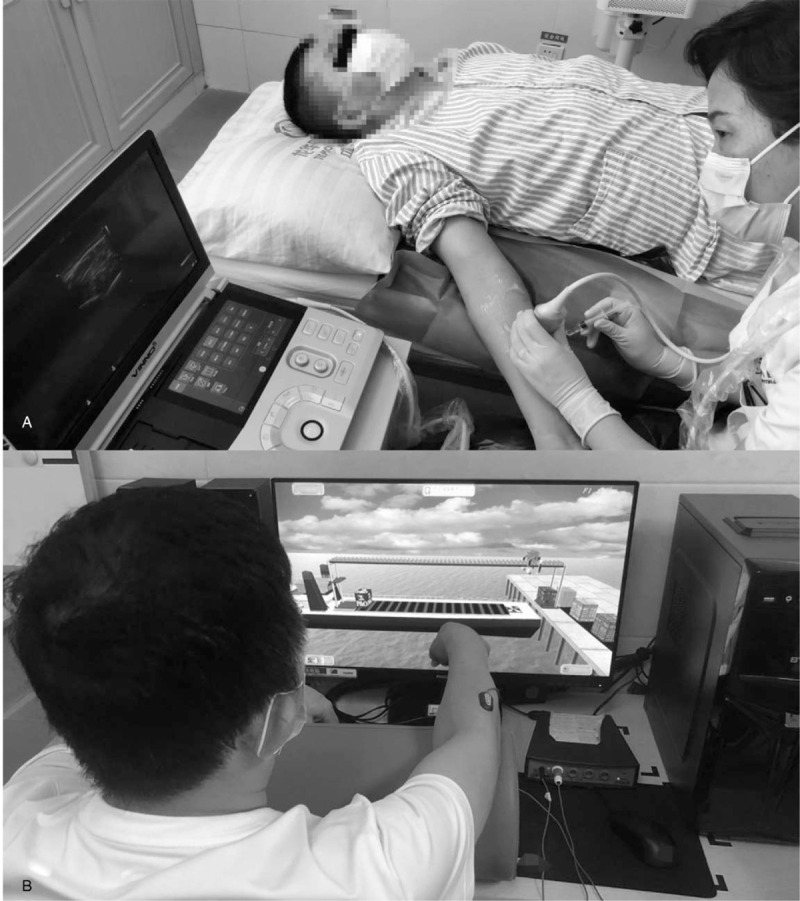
Botulinum toxin type A injection through ultrasound-guided (A), E-link biofeedback games training program (B).
